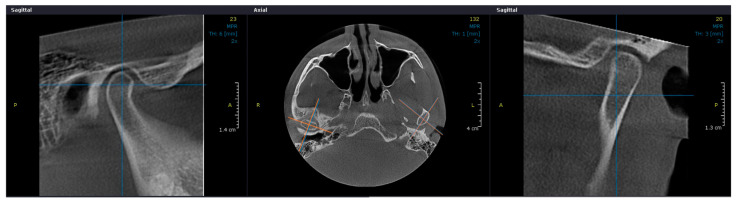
CBCT images selection for morphological assessment: the axial slice with the largest mediolateral diameter of the condylar head with a sagittal slice crossing in the middle of the distance between the most prominent points on medial and lateral poles and perpendicular to the coronal axis.
